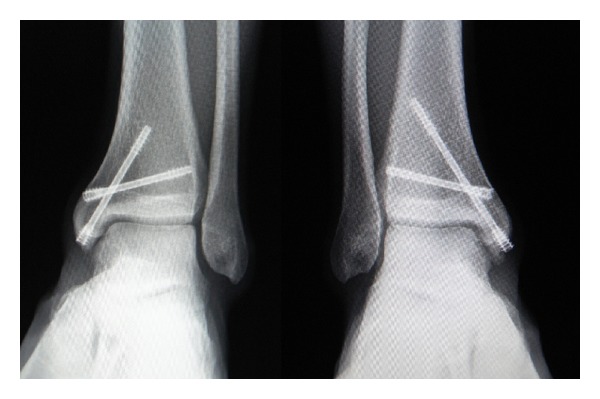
Anteroposterior radiograph at 4 years after surgery shows continued and complete bony healing in both ankles.
